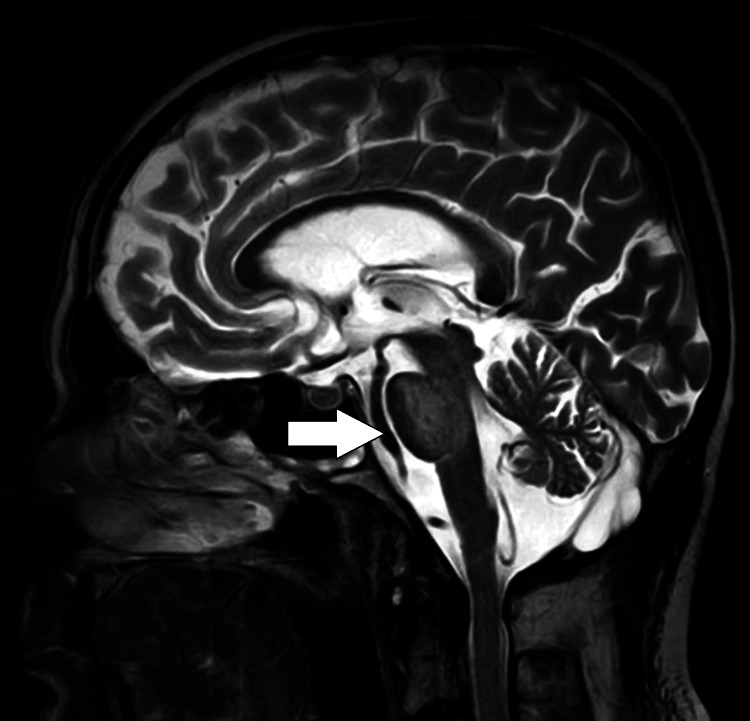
T2WI sagittal section of brain showing hyperintense lesion in pons T2WI: T2-weighted imaging
